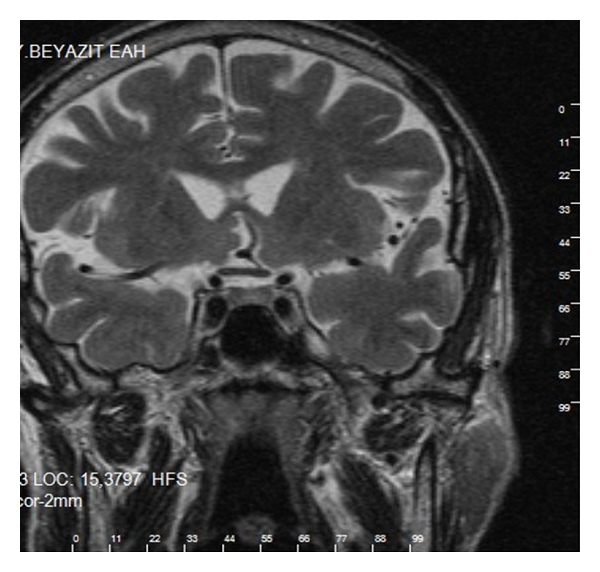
T2-weighted MRI demonstrated that 5 × 8 mm mass the has irregular borderline.
